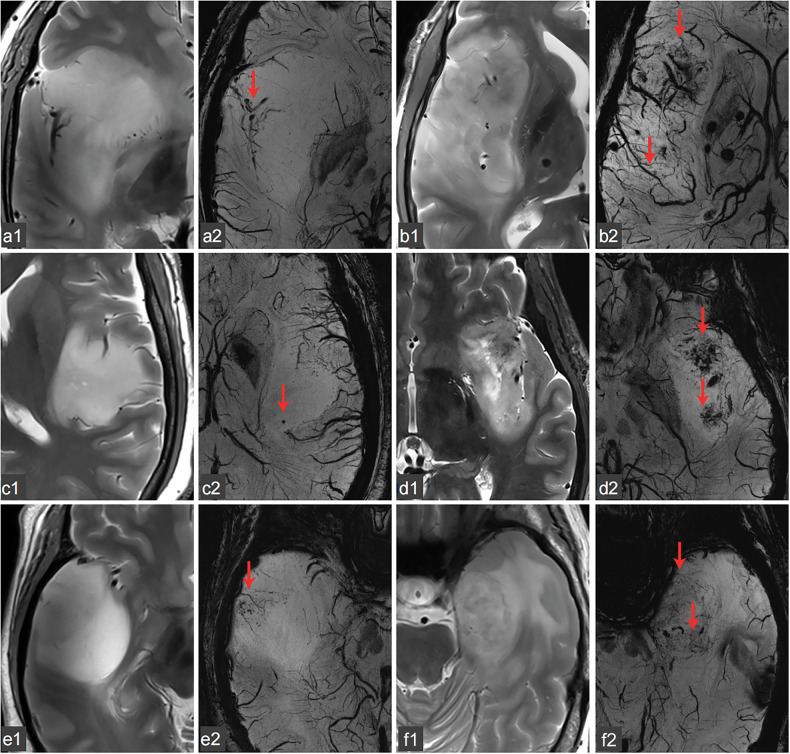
ITSS presentation is associated with the expression status of different molecular markers (as indicated by arrows). Demonstrated through two sequences: T2-weighted imaging (a1, b1, c1, d1, e1, f1) and SWI (a2, b2, c2, d2, e2, f2). a1, a2 Astrocytoma WHO III, low Ki-67 expression, ITSS 2; b1, b2 Oligodendroglioma WHO III, high Ki-67 expression, ITSS 4; c1, c2 Astrocytoma WHO II, no TERT promoter mutation, ITSS 2; d1, d2 Oligodendroglioma WHO II, TERT promoter mutation, ITSS 4; e1, e2 Astrocytoma WHO III, IDH1 mutation, no TERT promoter mutation, ITSS 2; f1, f2 Glioblastoma WHO IV, no IDH1 mutation, no TERT promoter mutation, ITSS 3
